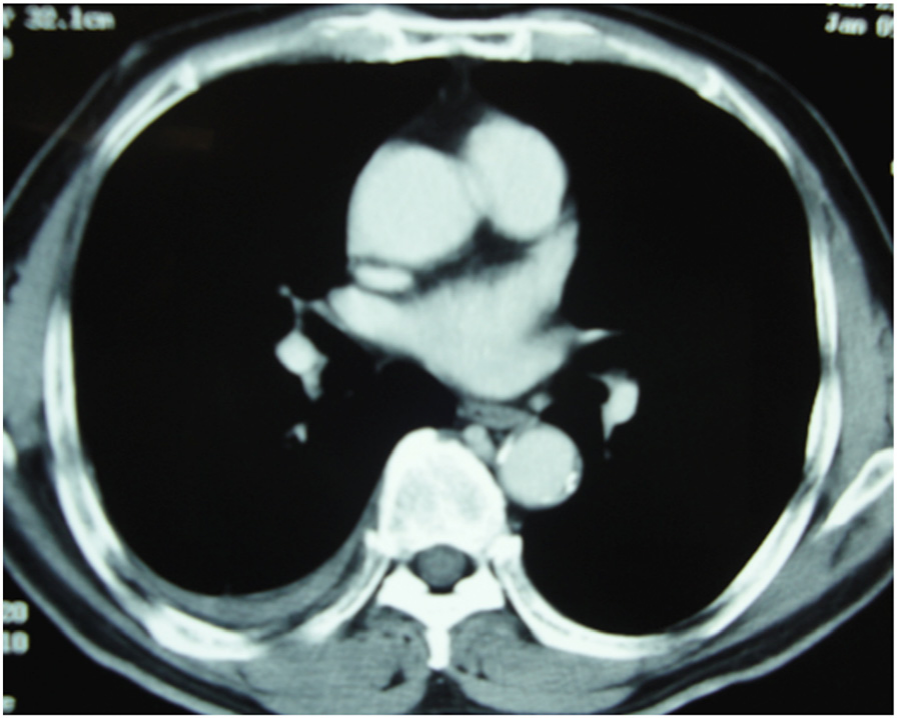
A computed tomography image at the time of complete response. One month after the treatment completion, the pleural thickening had completely disappeared. A small amount of right pleural effusion was observed, but has not increased since.
